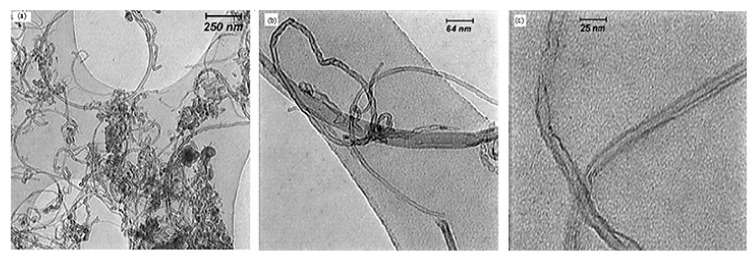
TEM images of as-prepared CNTs (a) catalyst concentration = 3 wt %, hydrogen flow = 240 cm^3^/min, and temperature = 900 °C; (b) catalyst concentration = 4 wt %, hydrogen flow = 120 cm^3^/min, and temperature = 1000 °C; (c) catalyst concentration = 5 wt %, hydrogen flow = 160 cm^3^/min, and temperature = 900 °C, by using ultrasonic bath.
